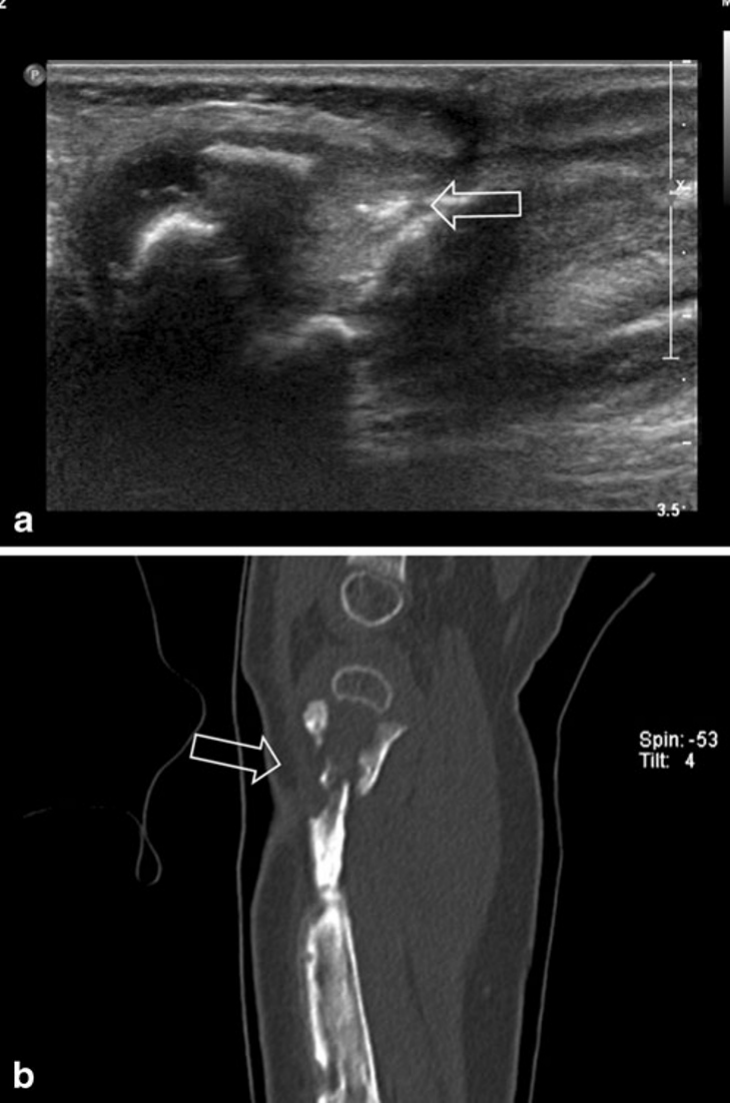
a A 3-month-old girl after treatment for osteomyelitis. In the follow-up, there was the suspicion of a sequester in the proximal metaphysis. Ultrasound was performed, which showed hyperechoic fragments in a surrounding fluid collection, suspicious for a small sequester (*arrow*). b CT confirmed the presence of a small sequester (*arrow*)
